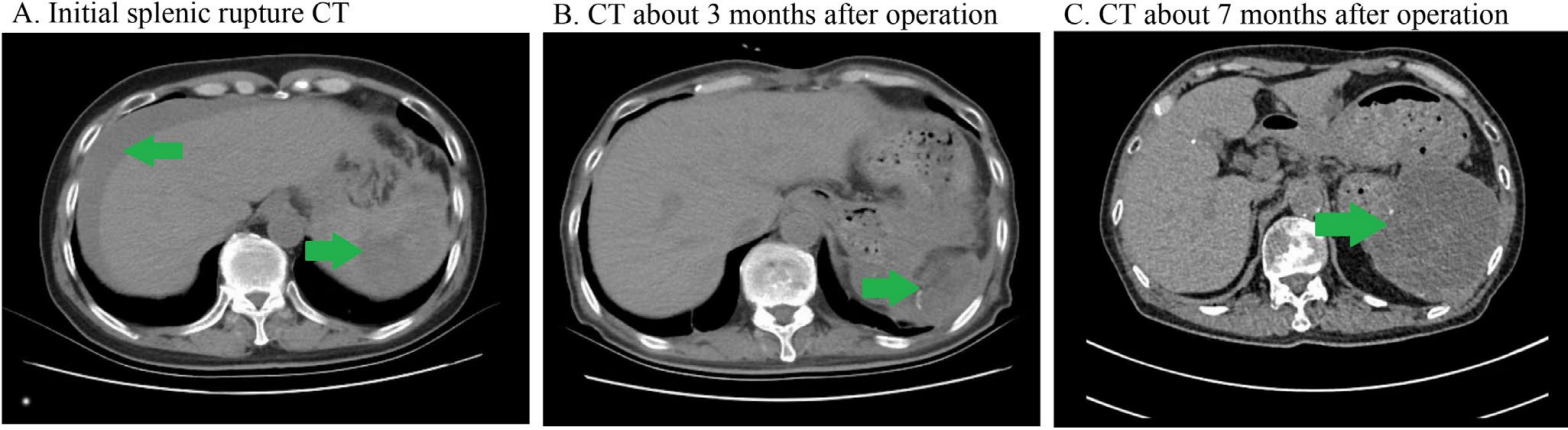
(A) The CT image of spontaneous splenic rupture. (B) The abdominal CT image at discharge after surgery. (C) Re‐examination of abdominal CT images after discharge for nearly 3 months.
